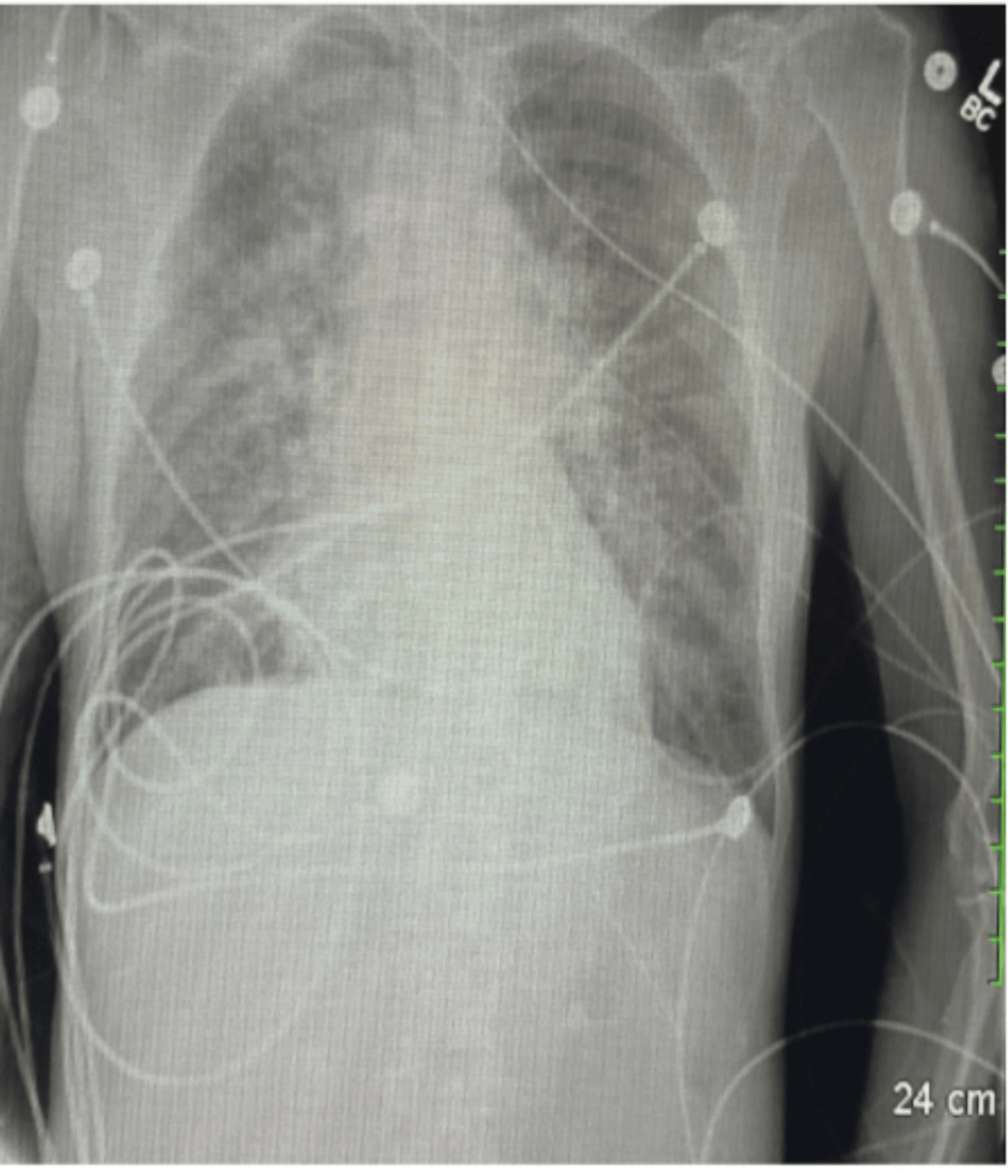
Repeat chest X-ray portable AP. Endotracheal tube is in satisfactory position. Bilateral interstitial opacities representing congestion. There is no evidence of pneumothorax or pleural effusion. Cardiomegaly was noted. Osseous structures appear to be intact, although partially visualized. AP: anterior posterior
